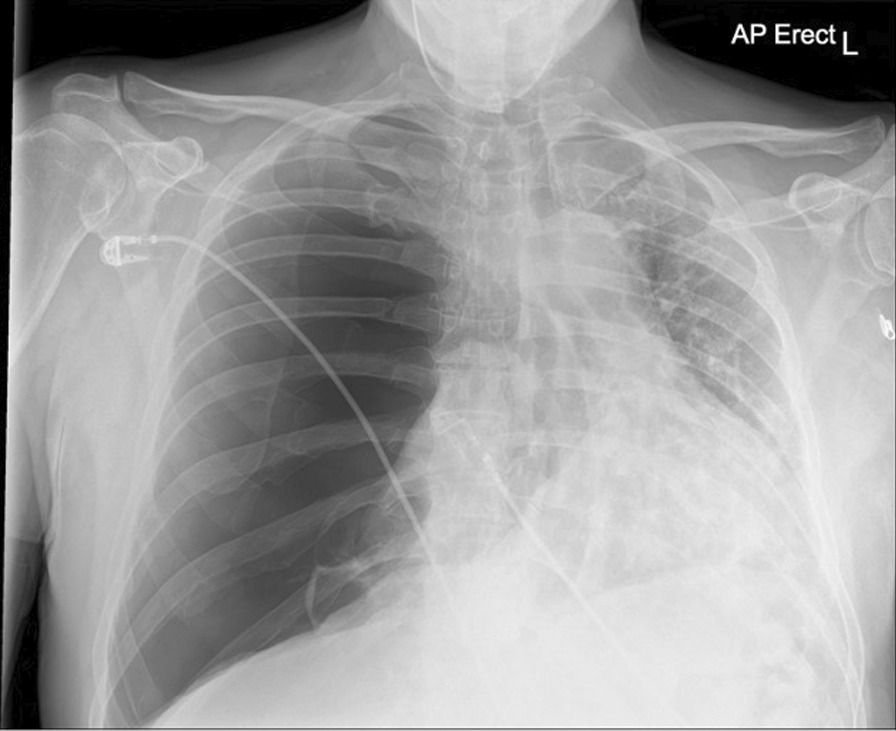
Chest x-ray of tension pneumothorax: Large right-sided tension pneumothorax causing deviation of the mediastinum to the left. The left lung is compressed
